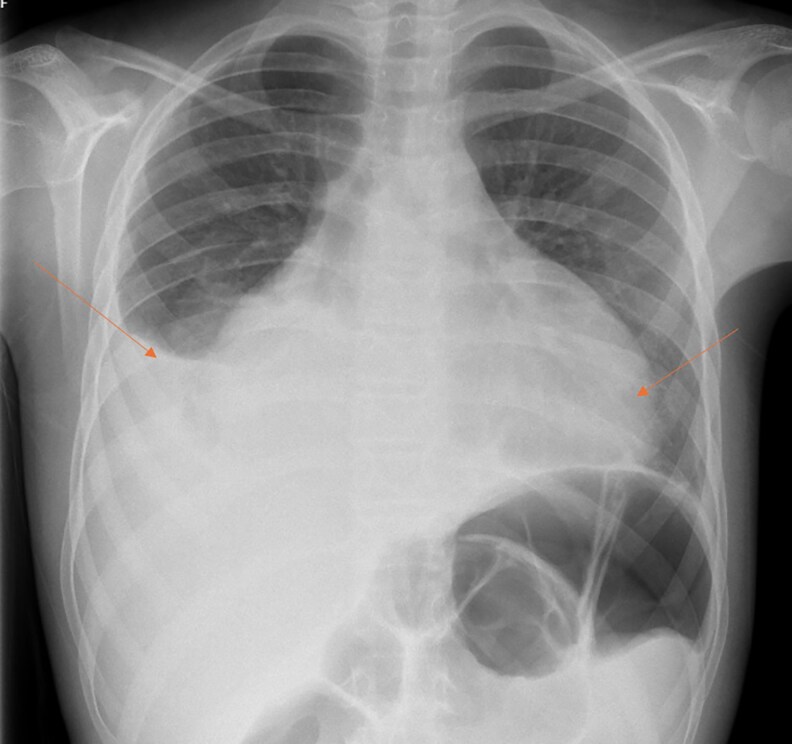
Chest radiograph taken the day of admission, depicting cardiomegaly and large right pleural effusion.
